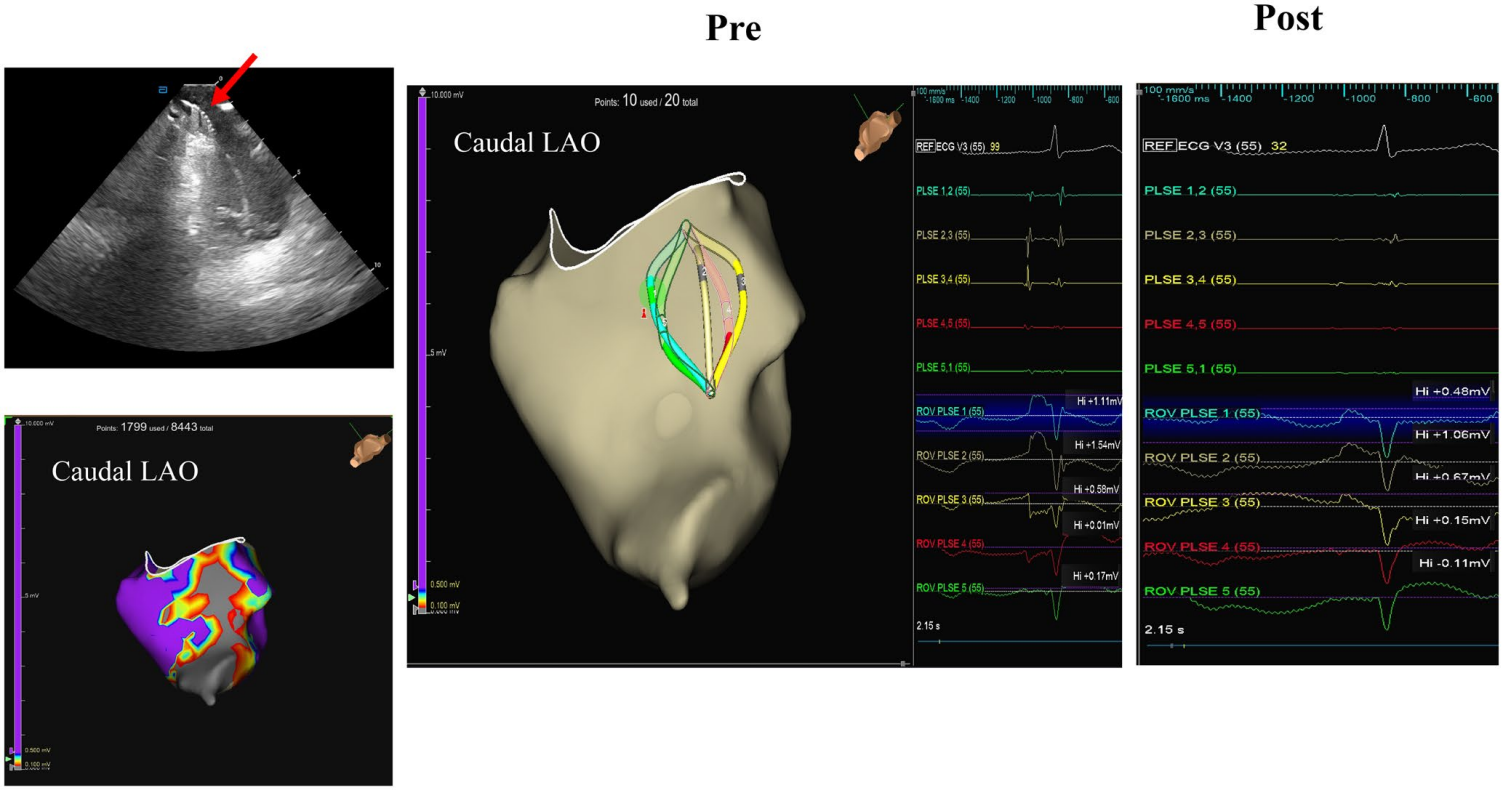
Tissue contact of the pulsed field ablation (PFA) catheter was confirmed by intracardiac echocardiography. The catheter was positioned along the cavotricuspid isthmus (CTI) line on 3D mapping, and unipolar electrograms from electrodes 1, 2, and 3 exhibited post‐P‐wave positive deflections. Bipolar electrograms demonstrated signal elimination after ablation, while the post‐P‐wave positive deflection persisted in unipolar recordings. The post‐ablation voltage map showed complete scar formation along the CTI line.
